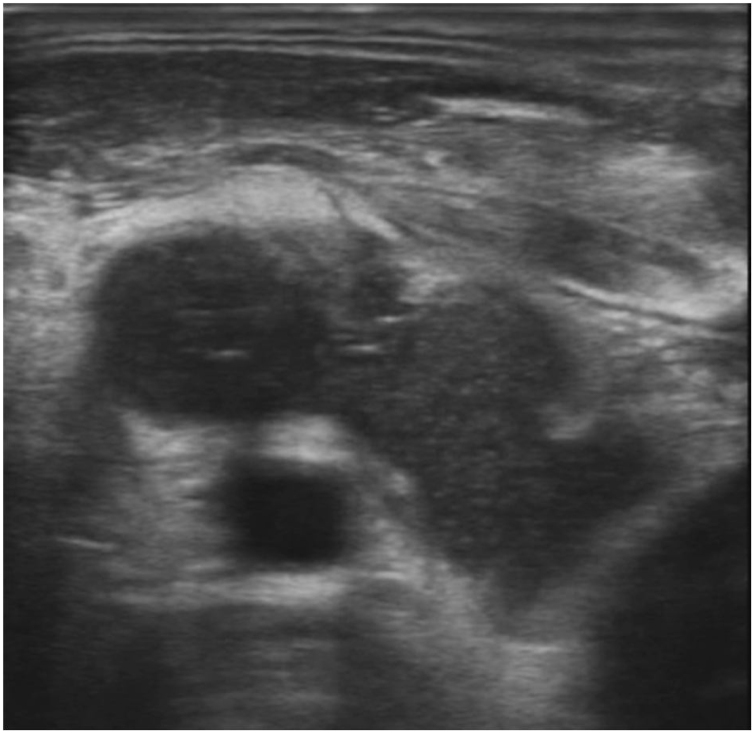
Ultrasonography finding showing right internal jugular vein invasion.
